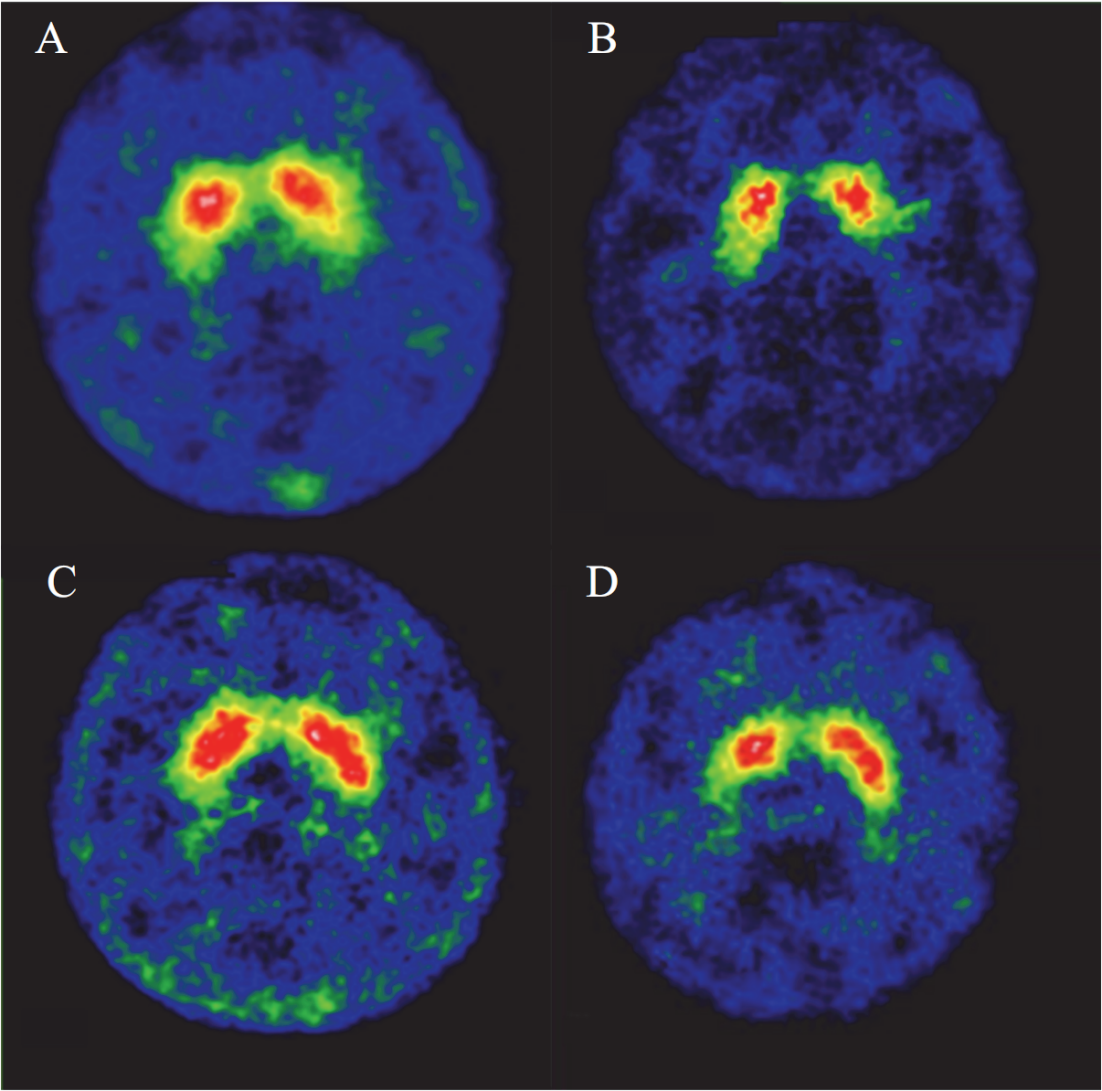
[^99m^Tc]TRODAT-1/SPECT images using the conventional setting for (A) a 34-year-old healthy female and (B) a 48-year-old male with PD, and using the optimal setting for (C) a 56-year-old healthy male and (D) a 45-year-old female with early PD.
